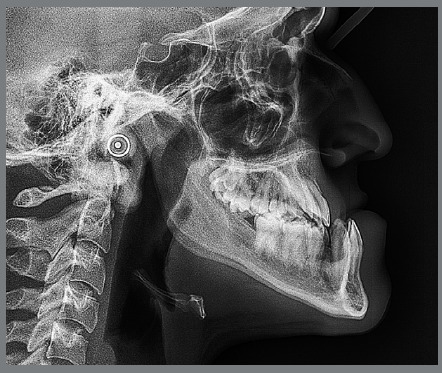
Initial lateral radiograph.
